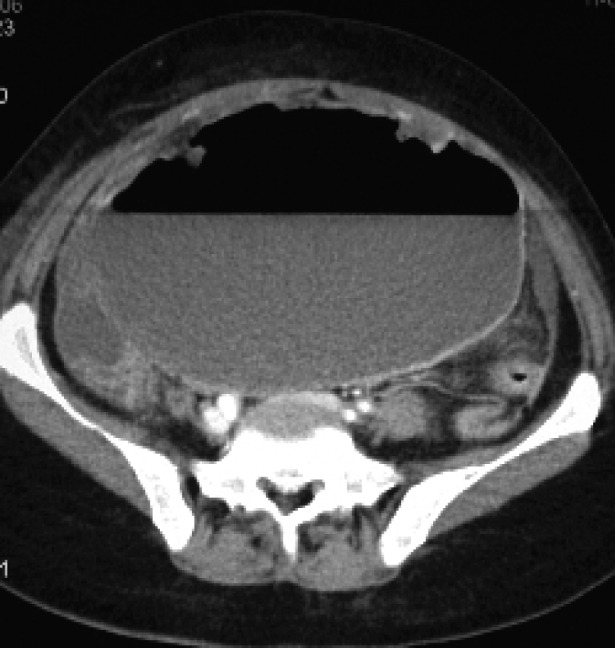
A postcontrast computed tomographic scan revealed a pelvic abscess with an air-fluid level.
